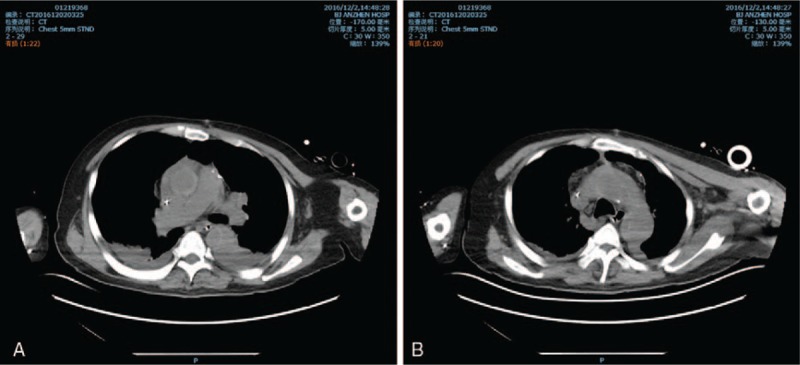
(A and B) The patient's postoperative aorta CT result, the CT aortography (CTA) was not practiced because of his renal dysfunction. Stanford A aortic dissection could be clearly confirmed by this CT scan.
